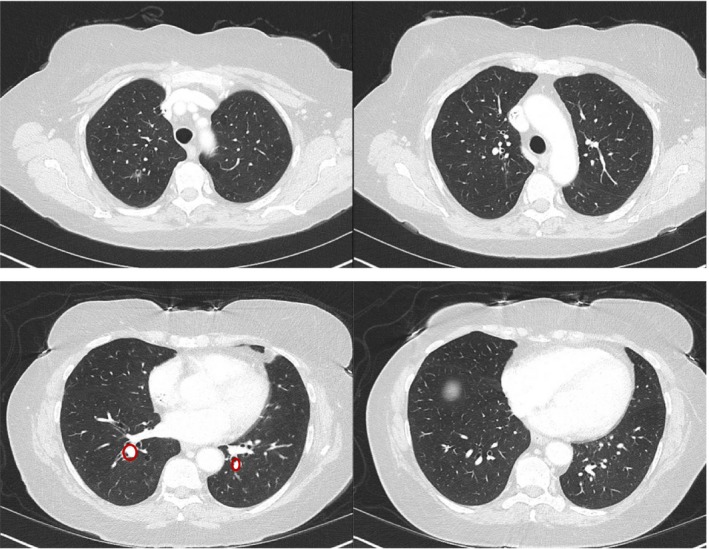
CT scans showing a near‐complete resolution of lung metastases after modified‐ docetaxel, cisplatin, and fluorouracil (DCF) chemotherapy
